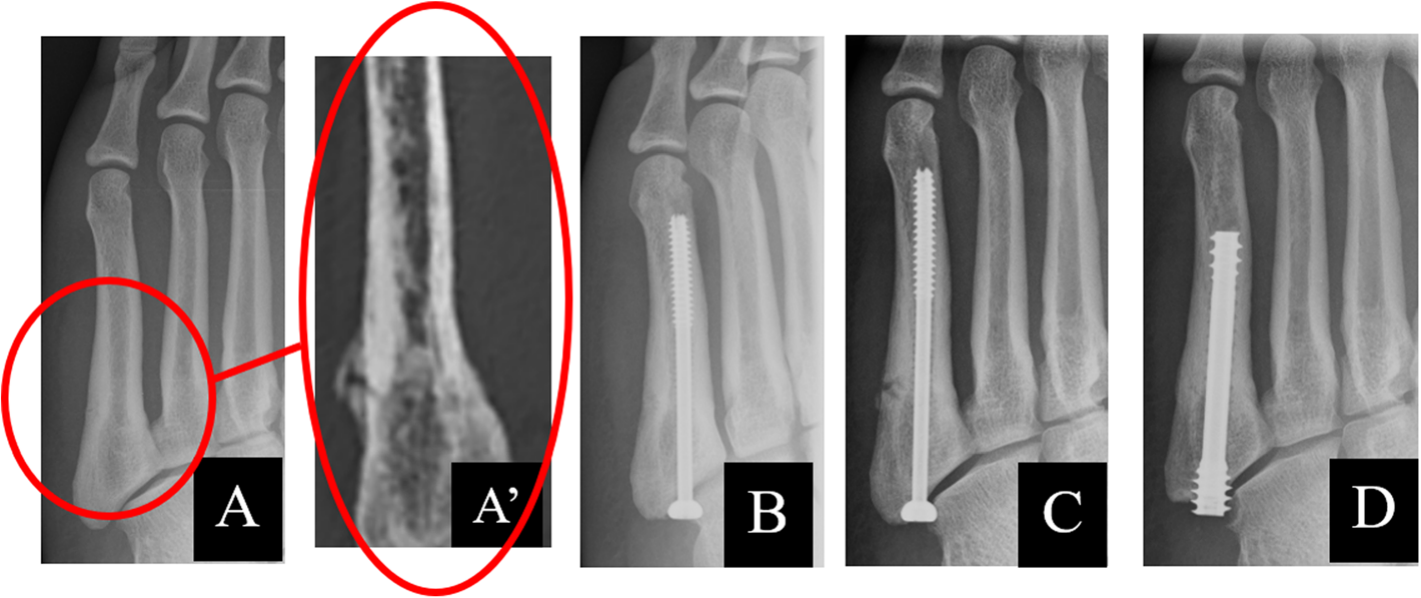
a Radiographs of the left foot before the primary operation. (A’) CT scan of the left foot before the primary operation. b Radiographs two months after the primary operation with a 4.5-mm-diameter screw. c Radiographs showing the re-fracture 3.5 months after the primary operation. d Radiographs showing bone union 3 months after re-operation
